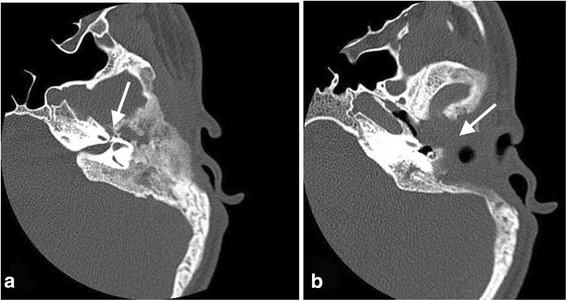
a Axial scan at the level of the labyrinth shows typical fibrous dysplasia-related ground-glass changes around the geniculate ganglion. b Axial CT scan at the level of the cochlea 1 year after surgery shows obliteration of the postoperative cavity
